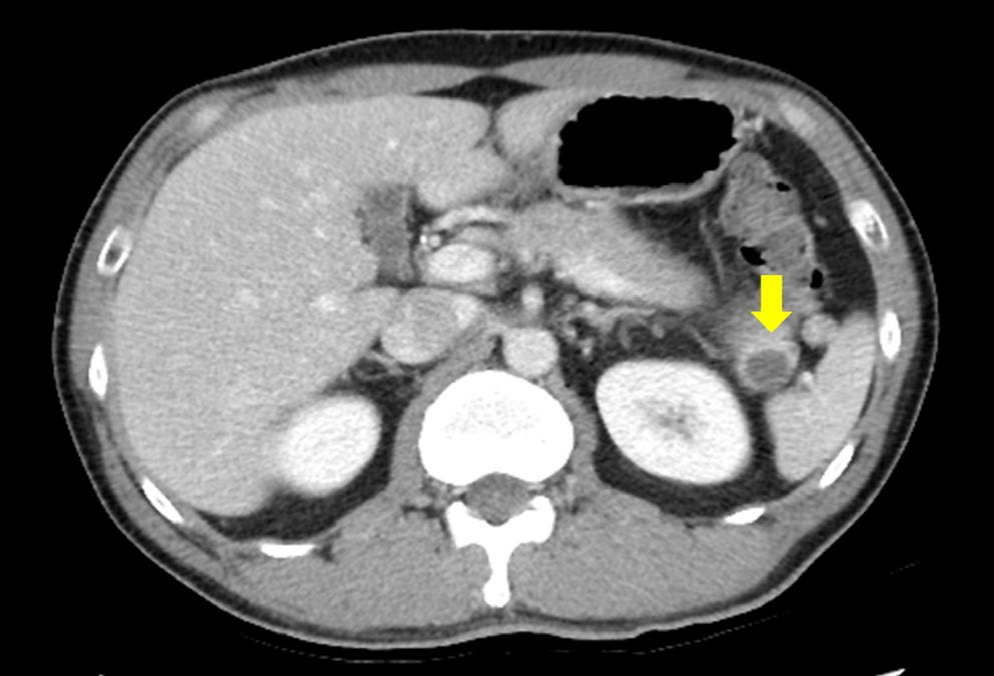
Computed tomography (CT): A cystic mass with a diameter of 15 mm in the pancreatic tail (arrow) is shown
